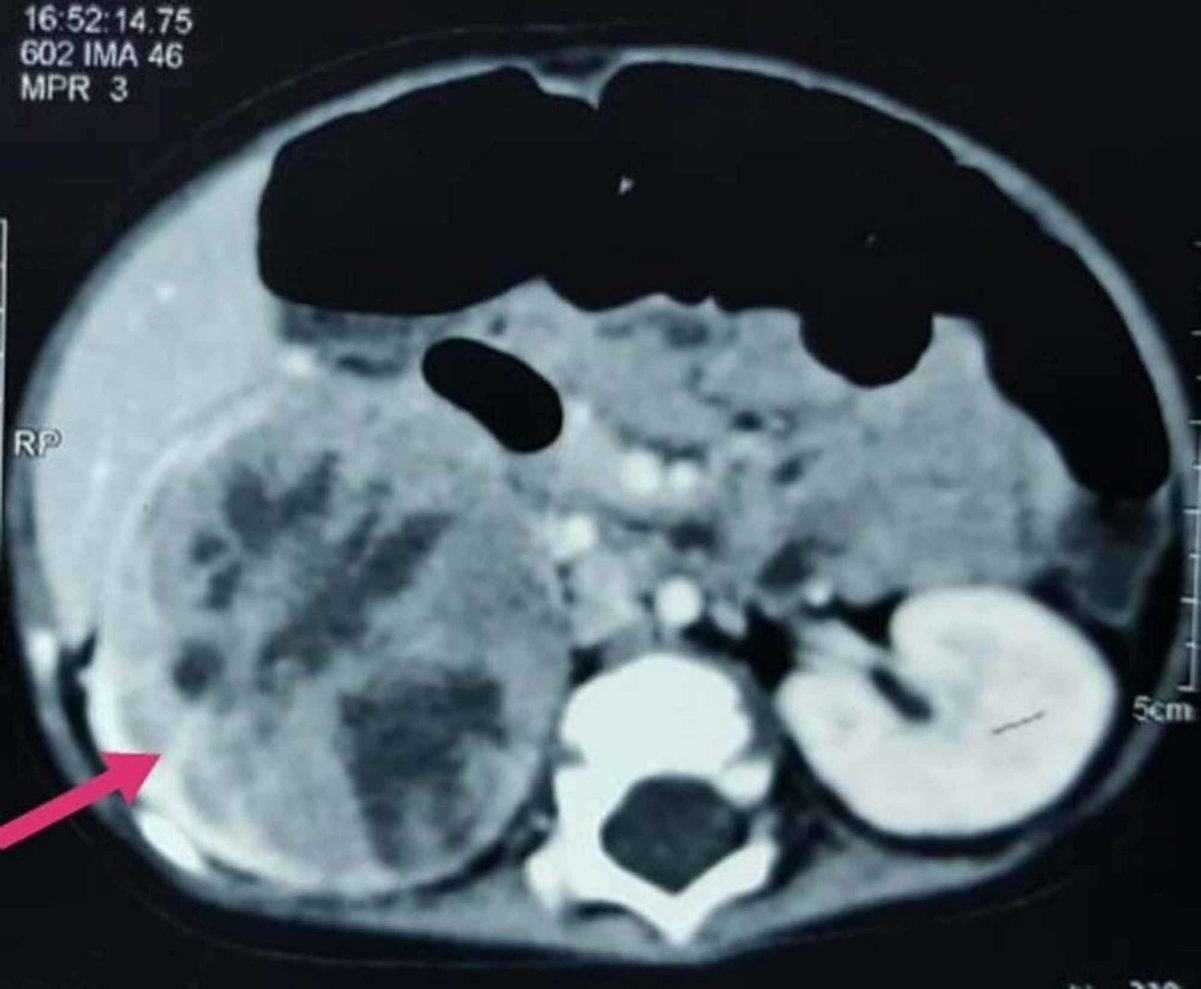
Computed tomography scan of the abdomen showing a huge lobulated mass arising from the right kidney with mild enhancement exhibiting multiple foci of necrosis
